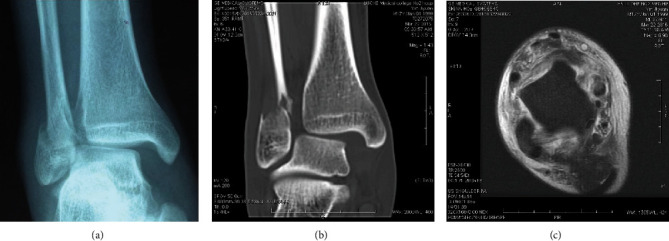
Preoperative imaging. (a) X-ray showed increased medial clear space (between the talus and medial malleolus). (b) CT demonstrated the distal tibiofibular joint separation with free fragments. (c) MRI showed full-thickness rupture of the deltoid ligament.
